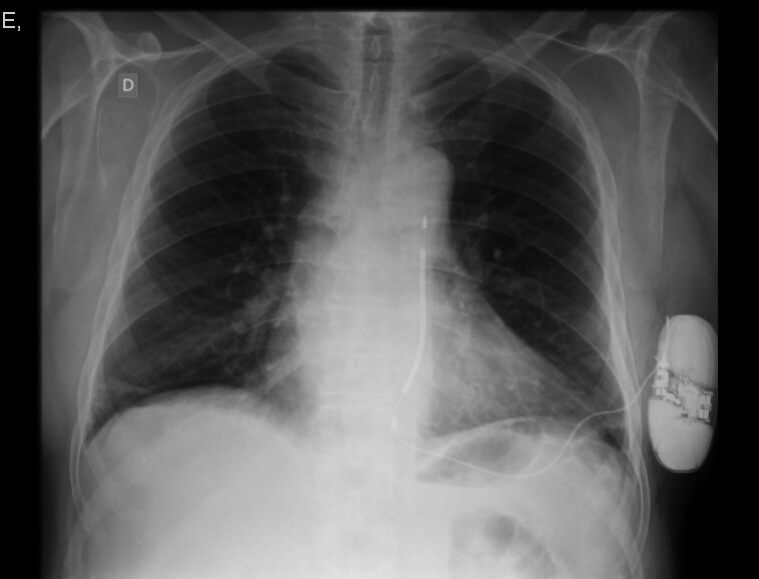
Postoperative posteroanterior (PA) chest radiograph
